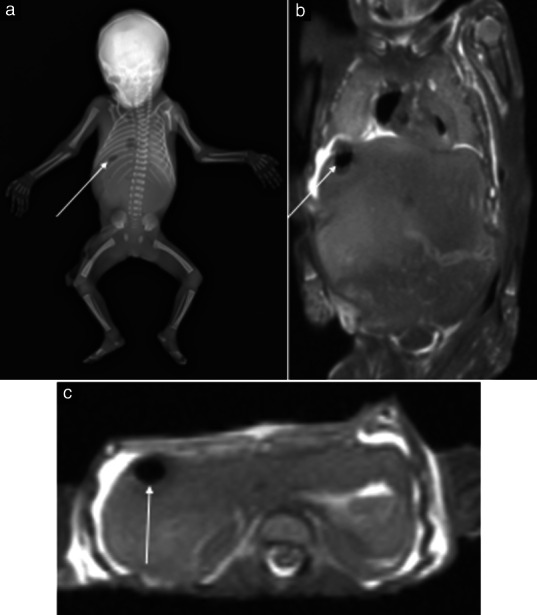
Normal postmortem appearance in miscarried 20‐week fetus which was diagnosed incorrectly with bowel perforation on postmortem magnetic resonance imaging (PM‐MRI). (a) Frontal view of whole‐body postmortem skeletal radiogram showing tiny locule of gas in right upper quadrant of abdomen (arrow). (b,c) Coronal (b) and axial (c) T2‐weighted PM‐MRI images of abdomen demonstrating small locule of gas (arrow); this was thought to represent bowel perforation, of which there was no evidence at autopsy.
